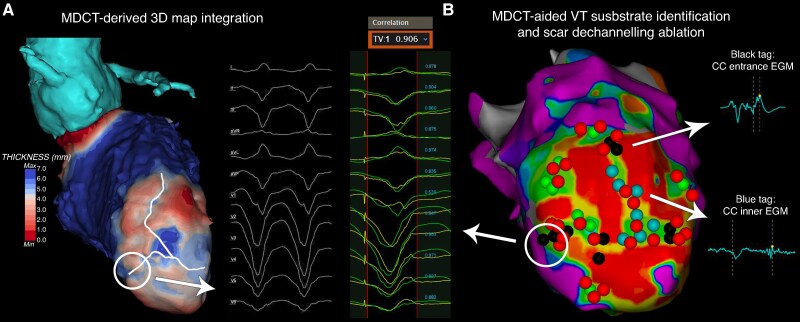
Multi-detector computed tomography-aided endocardial VT ablation in a post-myocardial infarction patient with an antero-apical scar. (*A*) Multi-detector computed tomography-derived LVWT map integration into the navigation system and imaging-aided VT exit localization according to pacemapping. (*B*) Voltage EAM rendering and VT substrate ablation according to scar dechannelling technique, with entrance, inner channel points, and HSC-EGMs ablation. CC, conducting channel; EGM, electrogram; MDCT, multi-detector computed tomography; VT, ventricular tachycardia.
